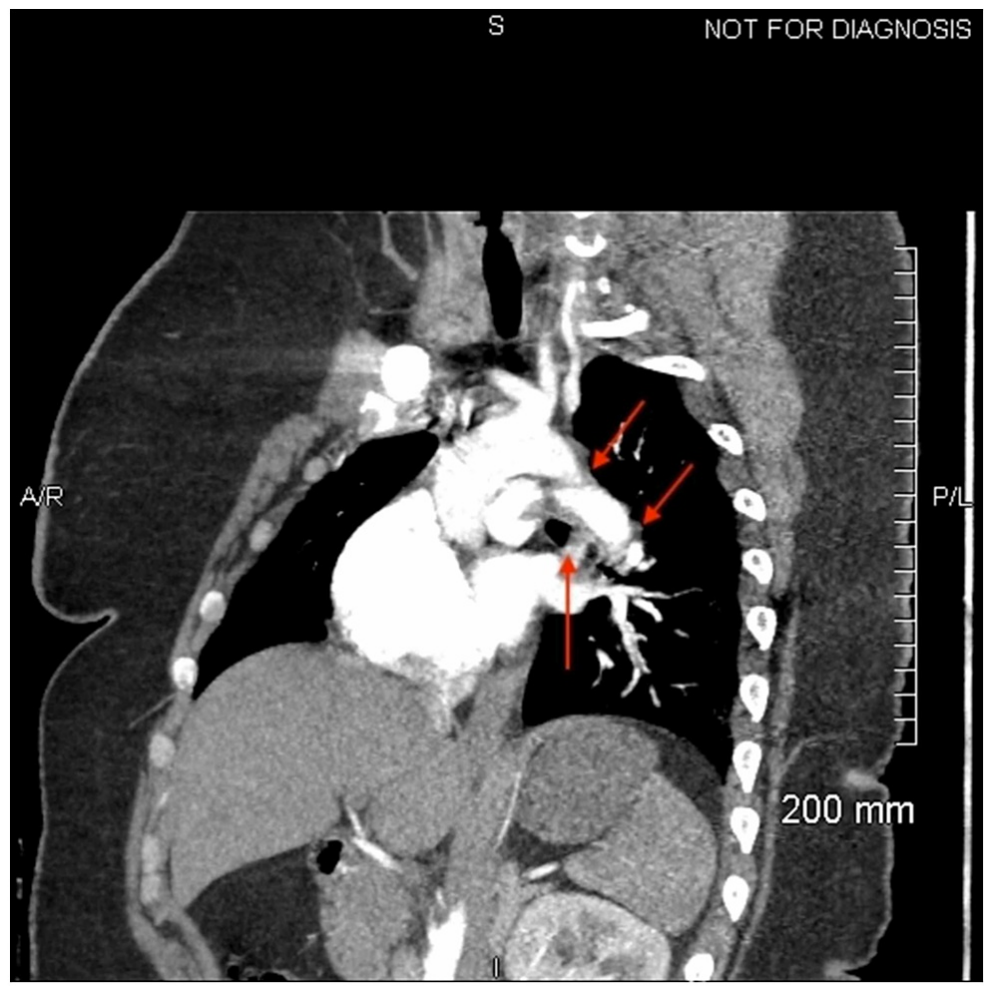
Sagittal view of the CT angiogram displaying a pulmonary embolism. The red arrows indicate the location of the embolis.
